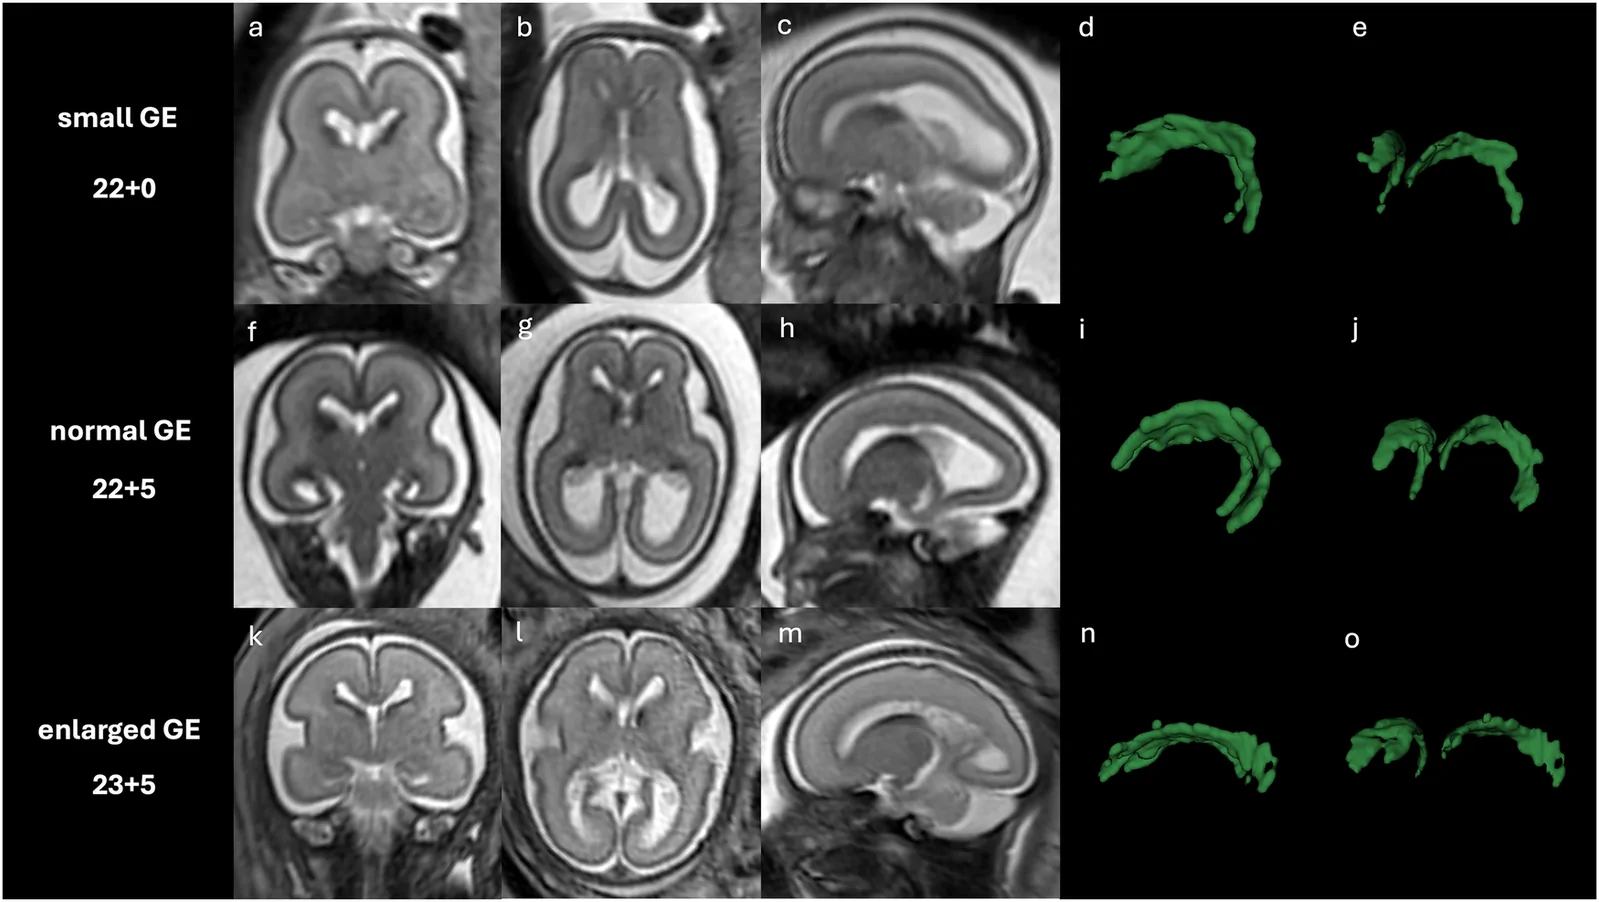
MRI super-resolution reconstruction and three-dimensional reconstruction depict the difficulty of subjective assessment of the ganglionic eminence. Too small ganglionic eminence (GE): T2-weighted MRI super-resolution reconstruction of a fetus at 22 + 0 gestational weeks in the coronal plane (a), axial (b), and paramedian sagittal plane (c). Three-dimensional reconstruction of the ganglionic eminence (GE) in lateral (d) and anterolateral oblique view (e). Normal-sized GE: T2-weighted MRI super-resolution reconstruction of a fetus at 22 + 5 gestational weeks in the coronal plane (f), axial (g), and paramedian sagittal plane (h). Three-dimensional reconstruction of the ganglionic eminence (GE) in lateral (i) and anterolateral oblique view (j). Enlarged GE: T2-weighted MRI super-resolution reconstruction of a fetus at 23 + 5 gestational weeks in the coronal plane (k), axial (l), and paramedian sagittal plane (m). Three-dimensional reconstruction of the ganglionic eminence (GE) in lateral (n) and anterolateral oblique view (o)
